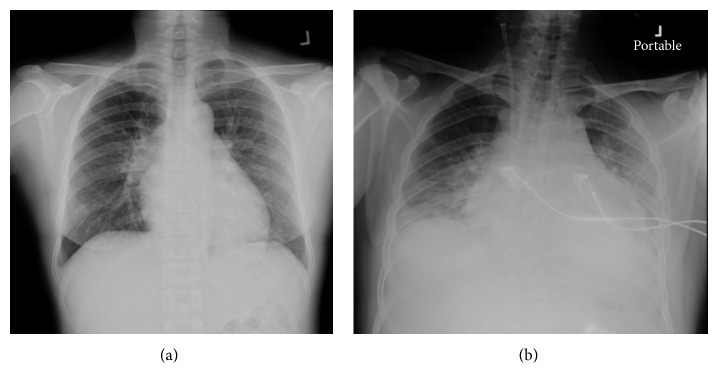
Chest X-rays: (a) prior to cytosine arabinoside; (b) day 3 of the first cycle of HIDAC.
